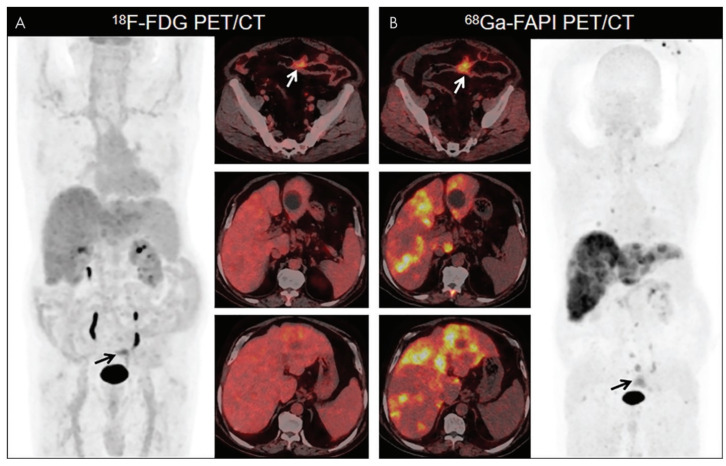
The uptake of ^68^GA-FAPI in the primary and metastatic lesions of colon cancer patients was higher than that of ^18^F-FDG, and the lesions were clearly shown. The black and white arrows show primary lesions of colon cancer. ^18^F-FDG PET/CT shows low to moderate metabolic activity in the primary colon and liver metastases ((A), left image: anterior maximum intensity projection image from ^18^F-FDG PET; right images: axial fused PET/CT images). ^68^Ga-FAPI PET/CT showed high uptake of primary lesions of colon and metastatic lesions of liver ((B), right image: anterior maximum intensity projection image from ^68^Ga-FAPI PET; left images: axial fused PET/CT images). Adapted from Ref. [59].
